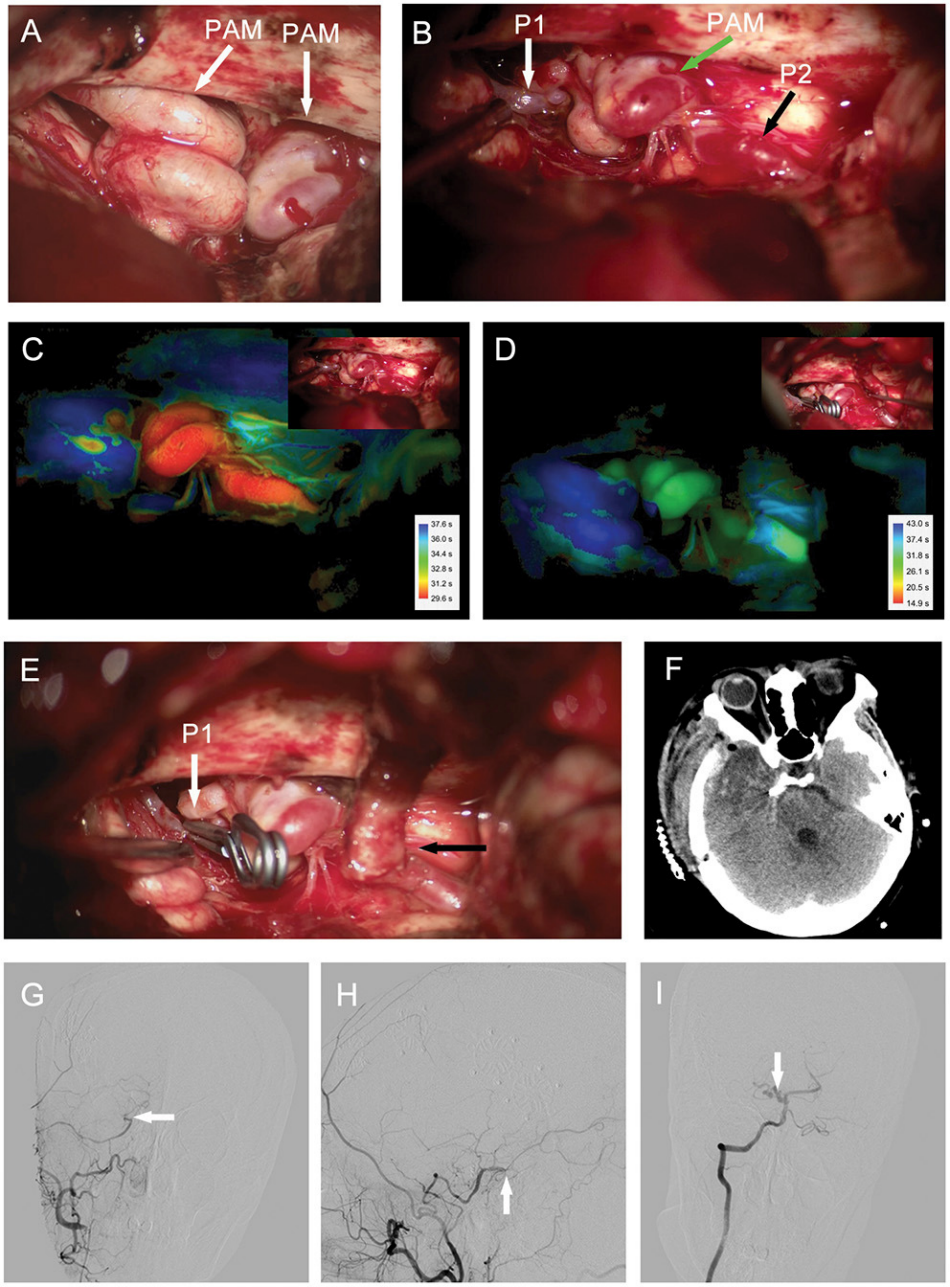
Case 2. (A,B) Intraoperative photograph showing (A) right ICA and (B) right P1 segment lesions composed of 2–3 tightly coiled loops (arrows). Intraoperative ICG video angiography analyzed with Flow800 revealing decreased perfusion in the lesion of P1 segment after proximal occlusion and bypass (D); compared to the image before proximal occlusion (C). Vascular lesion treated by [(E), white arrow] proximal occlusion of the P1 segment and [(E), black arrow] STA-P2 bypass. (F) Post-operative head CT operative region. Post-operative DSA showing bypass patency in [(G), arrow] anteroposterior and [(H), arrow] lateral views. [(I), arrow] Right vertebral artery angiograms demonstrating that the P1 segment lesion is smaller than the preoperative lesion in the anteroposterior view. CT, computed tomography; DSA, digital subtraction angiography; ICA, internal carotid artery; STA, superficial temporal artery.
